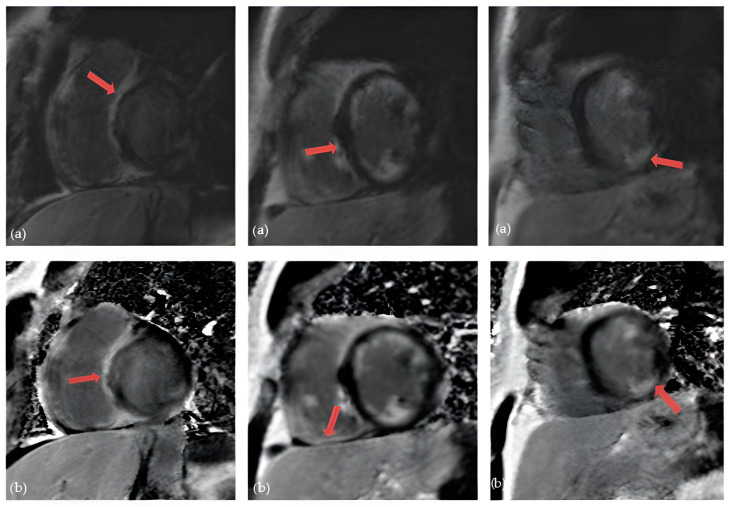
Cardiac magnetic resonance in a patient with cardiac sarcoidosis: (a) LGE MAG study, short axis: LGE in septum and inferior segments (marked with arrows); (b) LGE PSIR study, short axis: LGE changes in left and right ventricle, predominantly in septal segments (marked with arrows) (Avanto MRI, Siemens Healthcare GmbH, Erlangen, Germany, 1.5 T, CMR Lab University Clinical Hospital Center Bezanijska kosa, Belgrade, Serbia).
